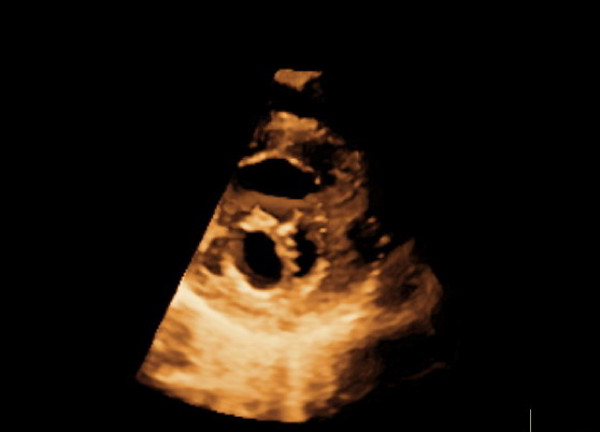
Parasternal short-axis view shows double-orifice mitral valve by three-dimensional echocardiography.
